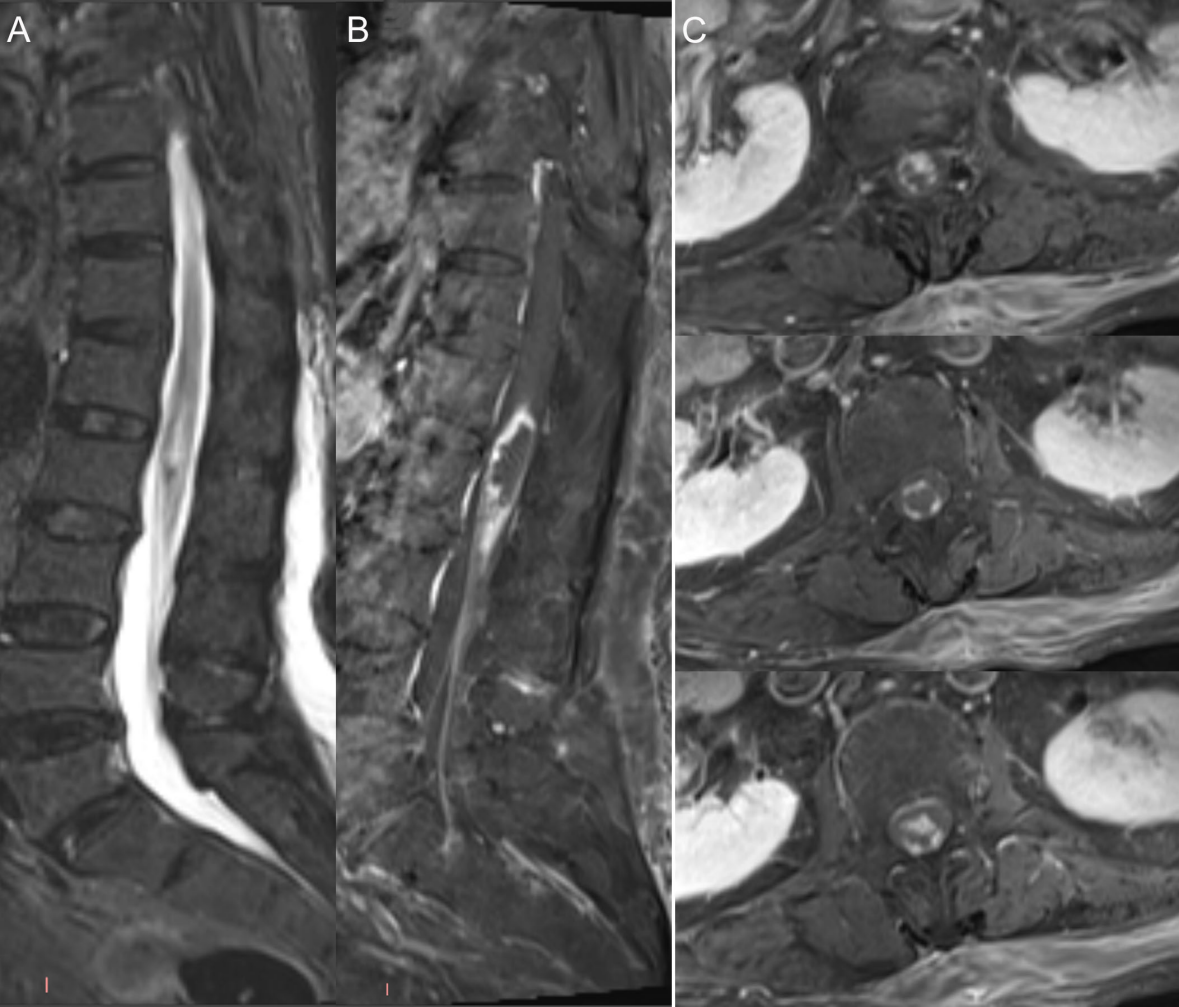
Spinal MRI findings demonstrating disease progression. Spinal MRI demonstrated an enlargement and enhancement of the spinal cord lesion, accompanied by extensive enhancement of the cauda equina nerve roots [(A) Sagittal T2WI; (B) Sagittal contrast-enhanced T_1_WI; (C) Transverse contrast-enhanced T_1_WI.
